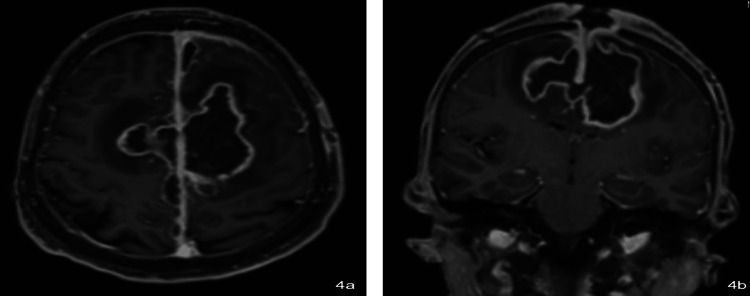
Postoperative day 10 MRI in (a) axial and (b) coronal planes showing bifrontal parafalcine abscess collection with surrounding edema and hemorrhage
